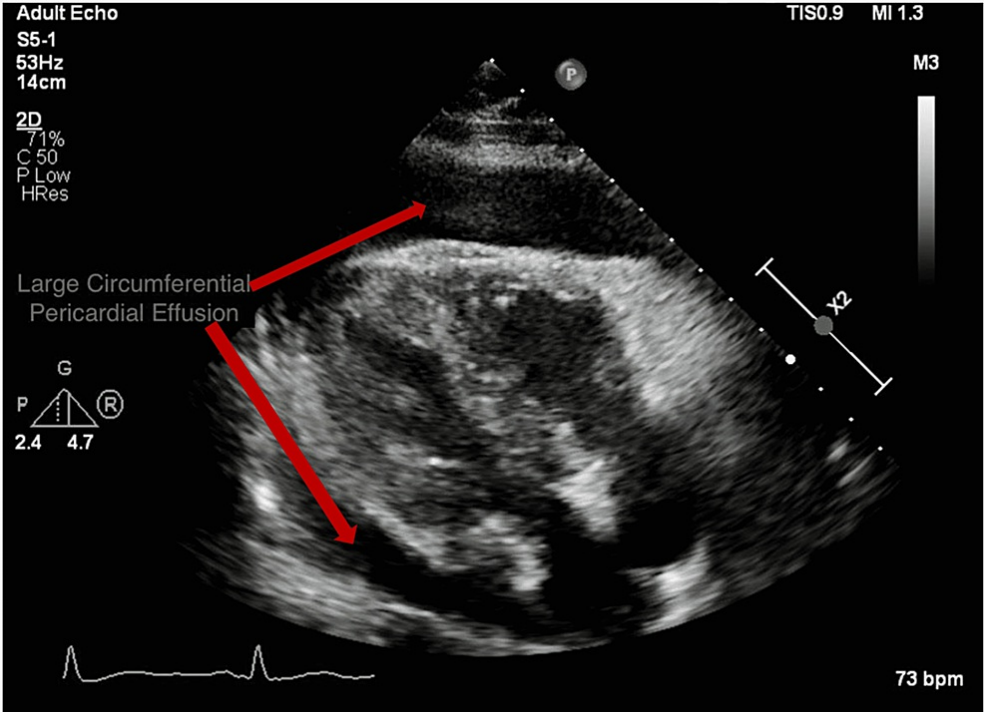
Transthoracic echocardiogram depicting large pericardial effusion.
